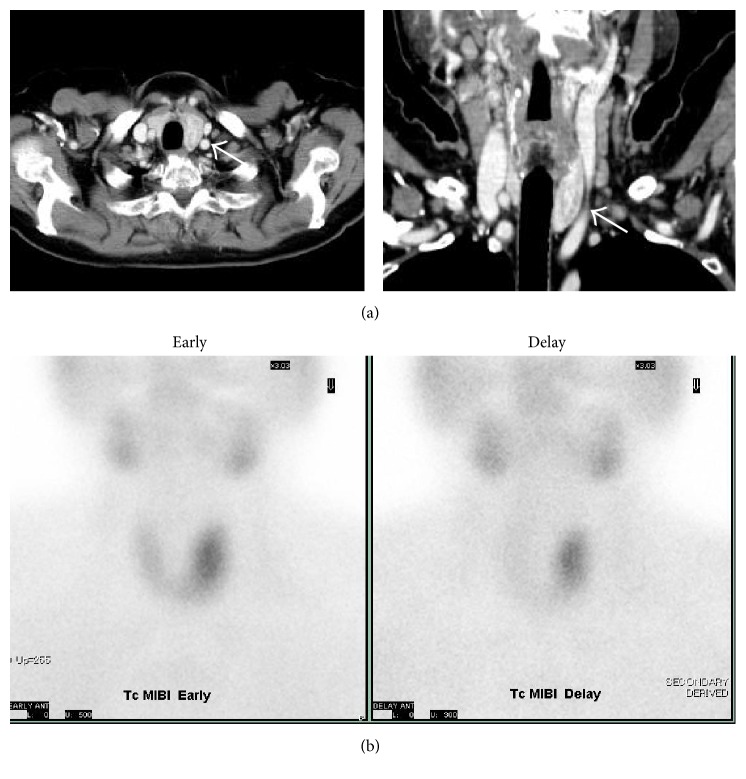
(a) Computed tomography of the neck. The scan with contrast enhancement shows a large heterogeneous soft tissue mass (28 mm transverse diameter), clearly defined, within the left thyroid lobe (white arrow). (b) ^99m^Tc-MIBI scintigraphy. Early and delayed scintigrams reveal focal accumulation of increased radiotracer uptake in the left lobe of the thyroid.
